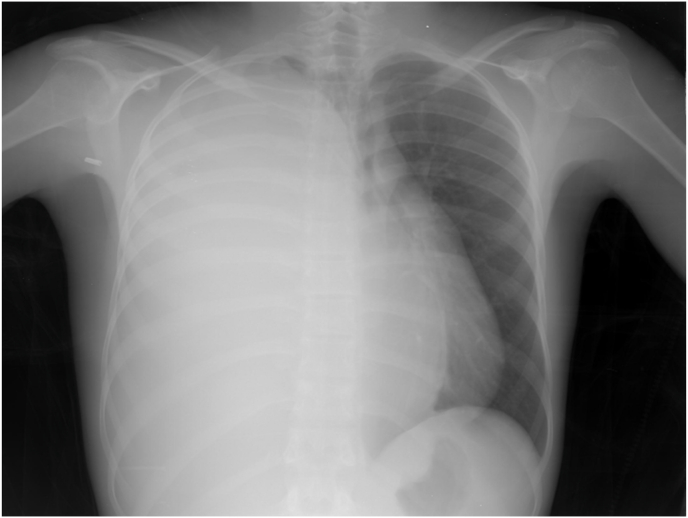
Patient's chest X-ray: a complete opacification of hemithorax on the right side.
